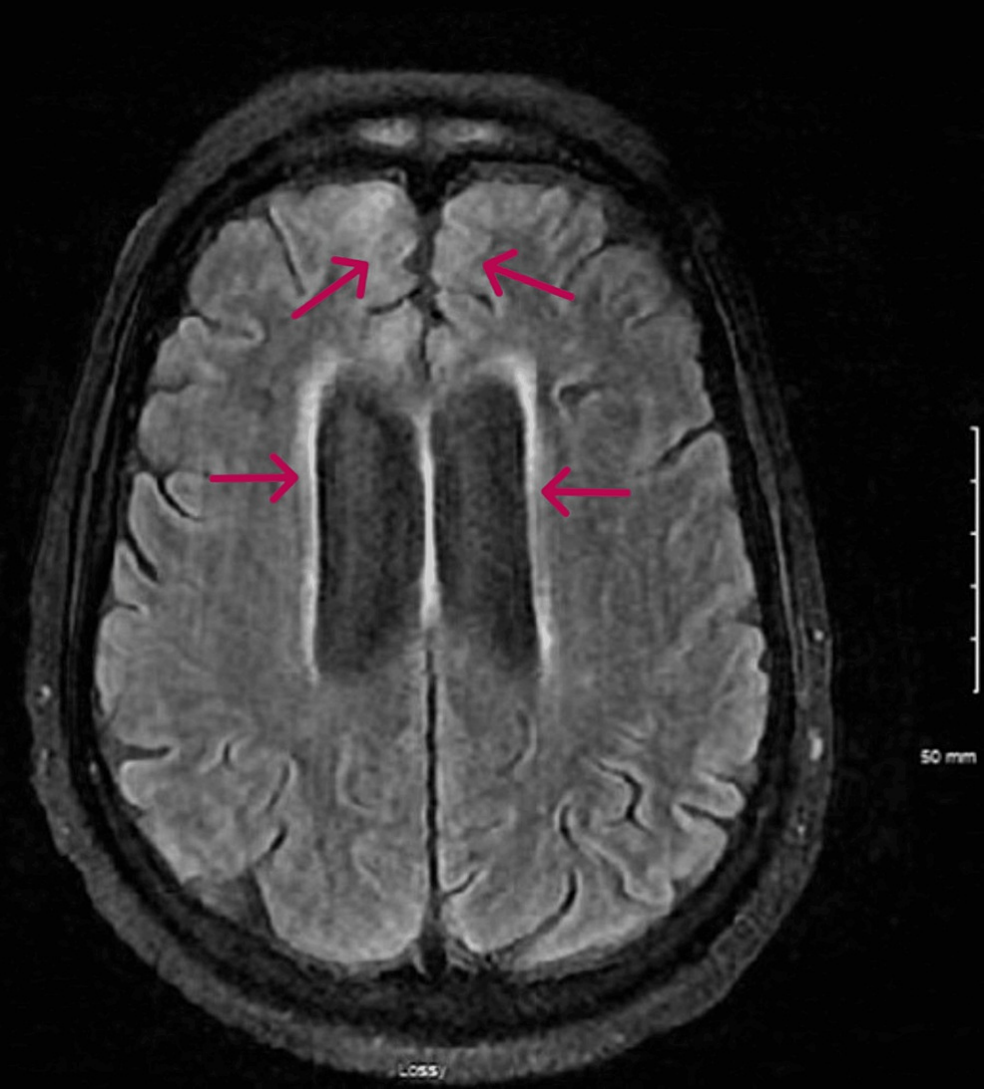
MRI Brain with persistent FLAIR signal hyperintensity in temporal lobes, particularly in the anterior horns, in the insular cortices, and the anterior inferior frontal lobes FLAIR: fluid-attenuated inversion recovery
